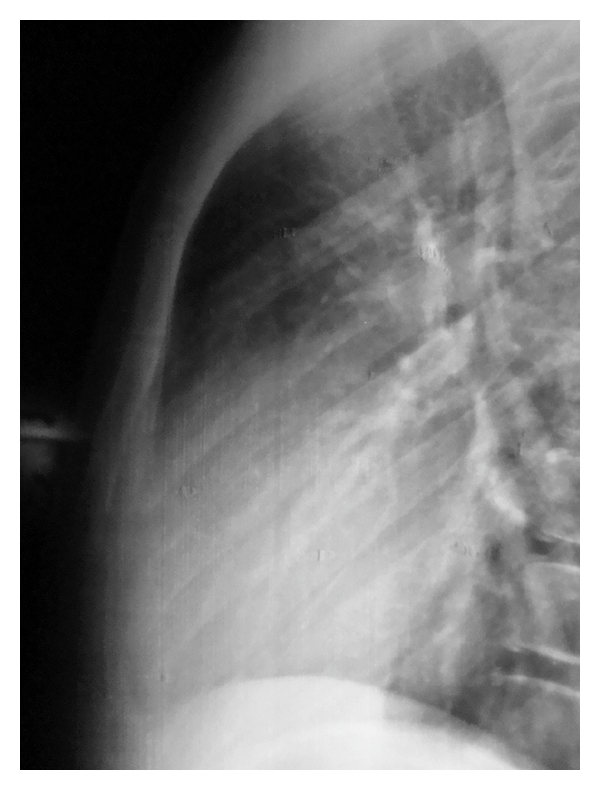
Lateral chest X-ray after reduction of the dislocated sternum.
